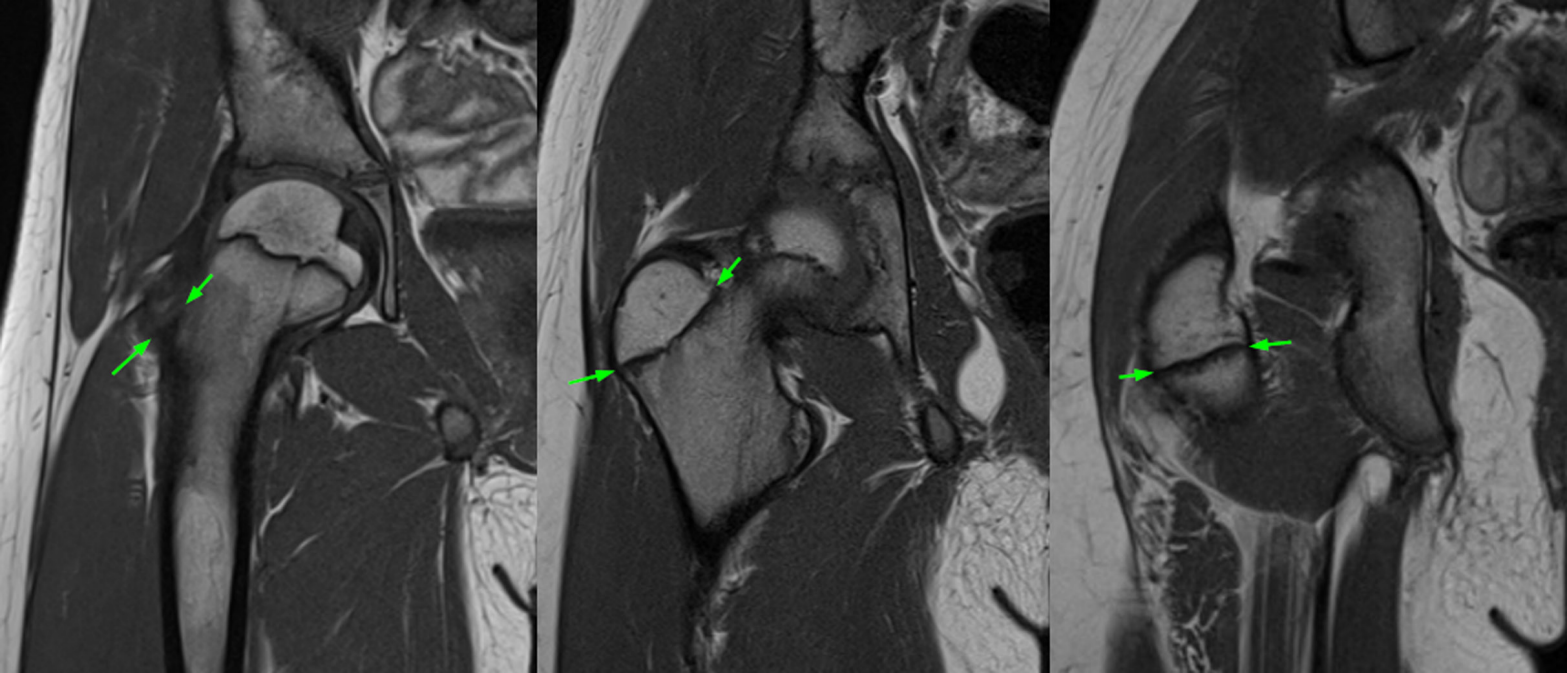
Magnetic resonance imaging, T1 sequence, of the right hip of a 10‐year‐old girl. The arrows mark the lateral and medial margins of the apophyseal plate. In the ventral and medial part of the apophysis (left and middle images), the apophyseal plate is aligned parallel to the femoral neck. The image on the right shows the dorsal aspect of the apophysis with a more horizontally oriented growth plate.
